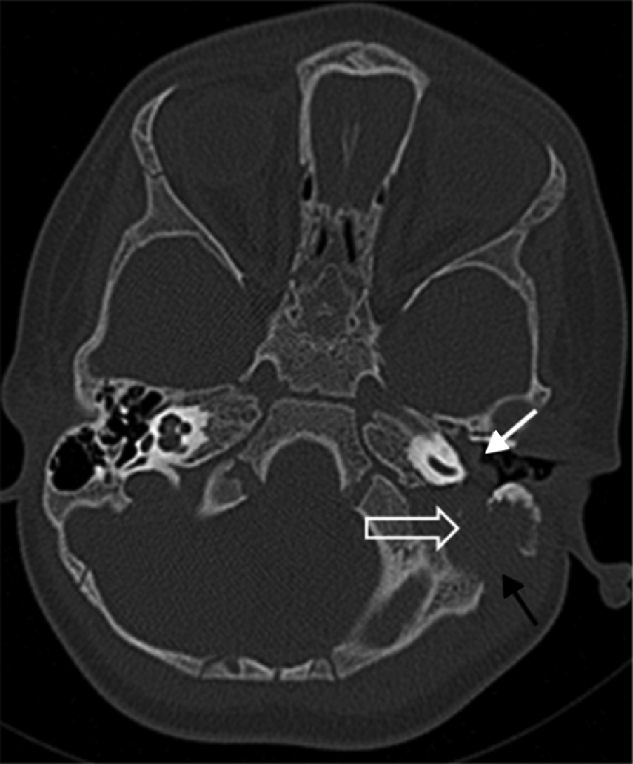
Axial computed tomography image at bone window shows a mass (open arrow) within the left mastoid air cells with an extension into the middle ear cavity (white arrow). Note the associated cortical erosion at the mastoid bone (black arrow).
